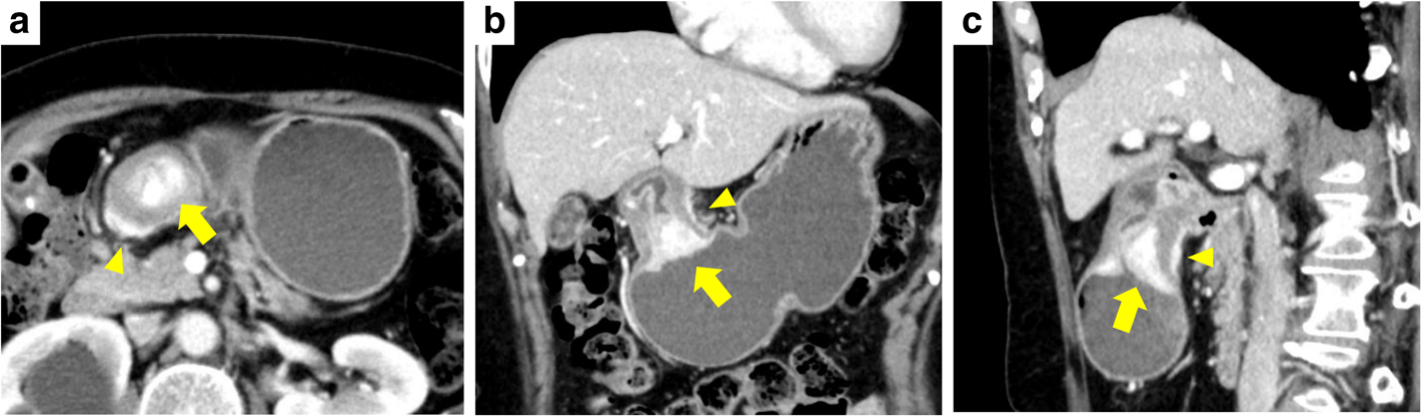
Computed tomography (CT) findings. a Axial view. b Coronal view. c Sagittal view. CT image showing a hyper-enhancing tumor in the submucosa of the prepyloric antrum (*arrows*). CT showing a thickening of the muscularis propria layer and a hyper-enhancing lesion in the subserosa of the gastric antrum (*arrow heads*)
